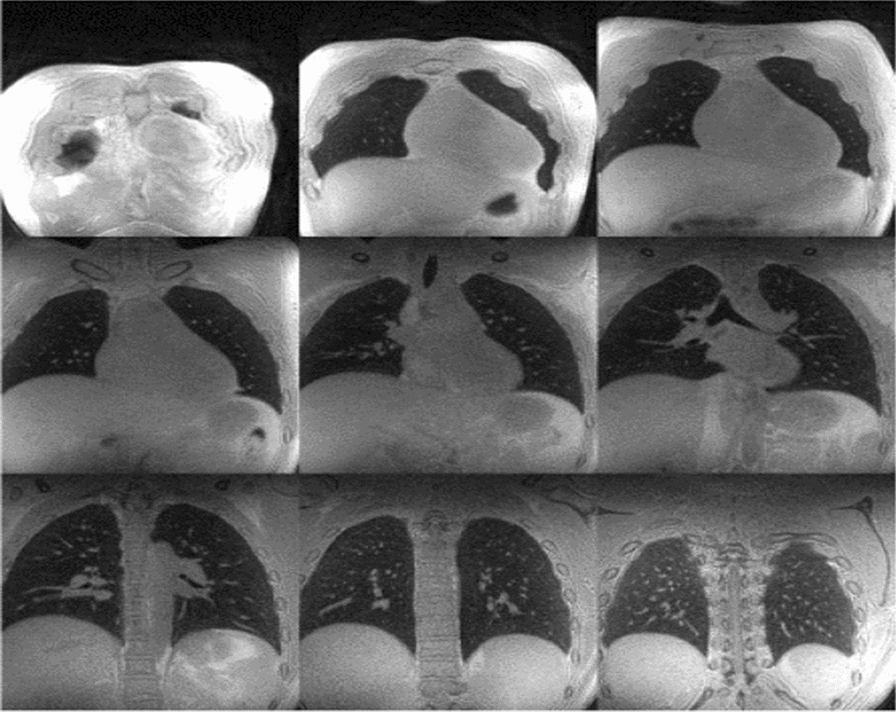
Coronal planes of UTE MRI and ZTE MRI of the lungs
